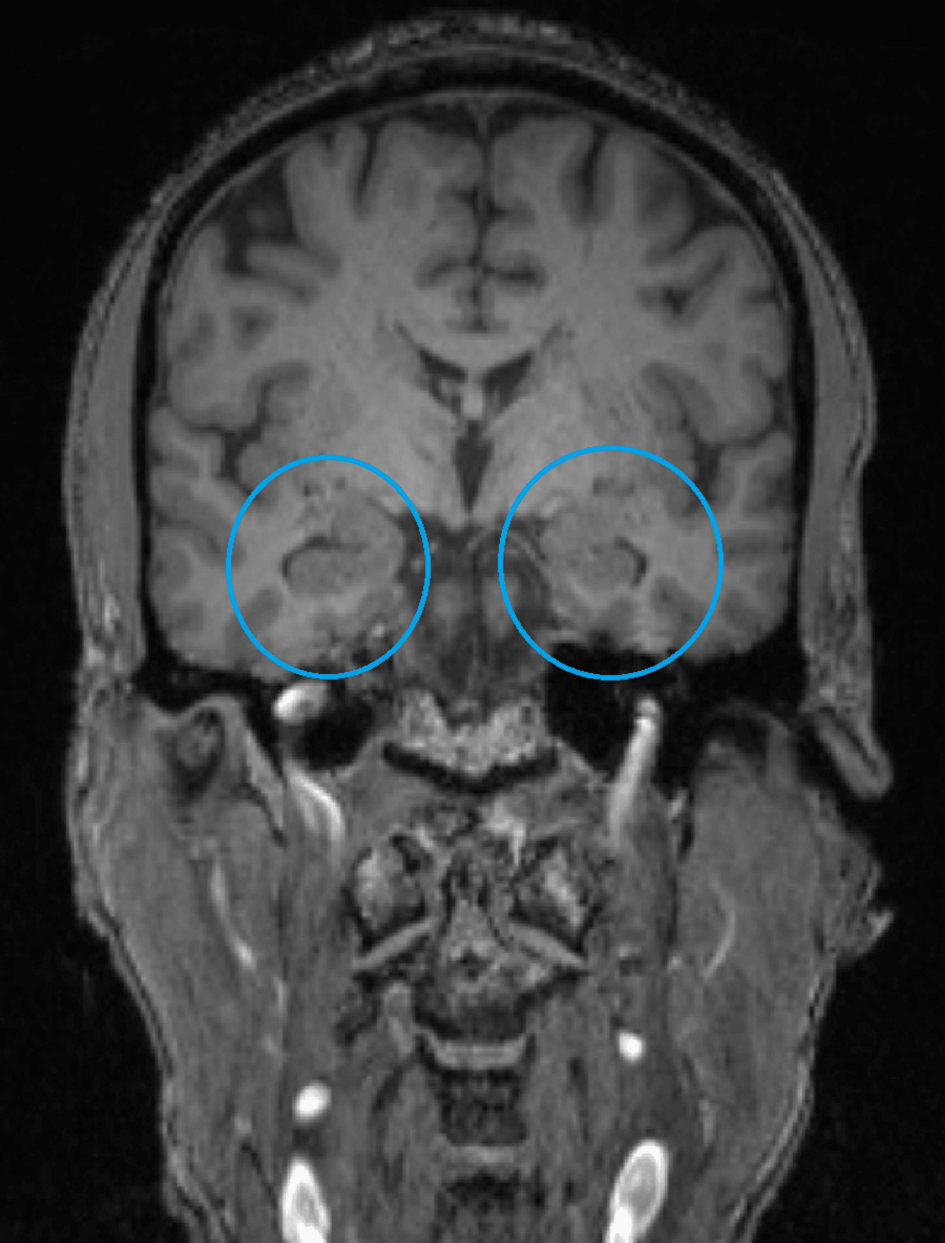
Coronal MRI No significant atrophy. Normal-sized hippocampus/entorhinal cortex volume (blue circle).
